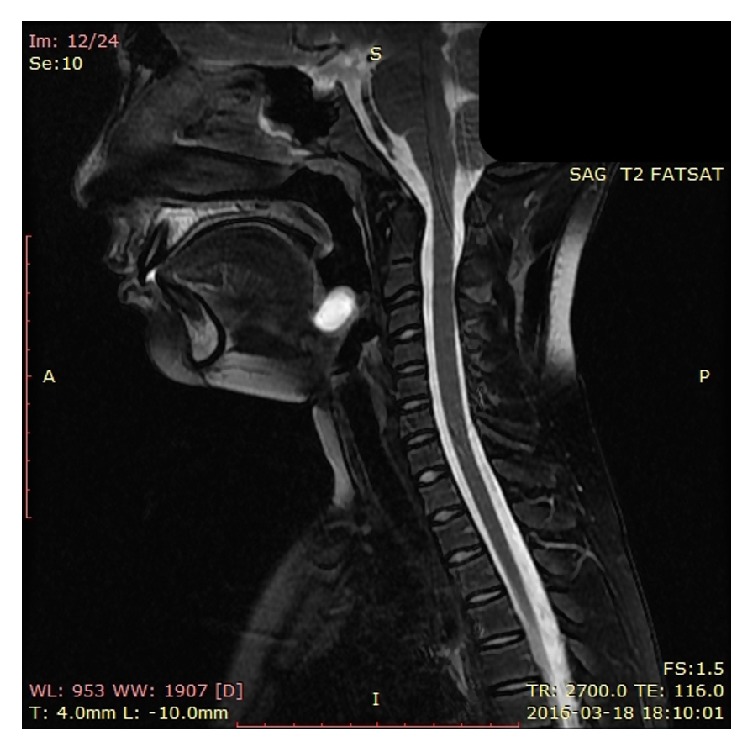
The recurrent lingual TGDC in 11-year-old boy in MR imaging.
